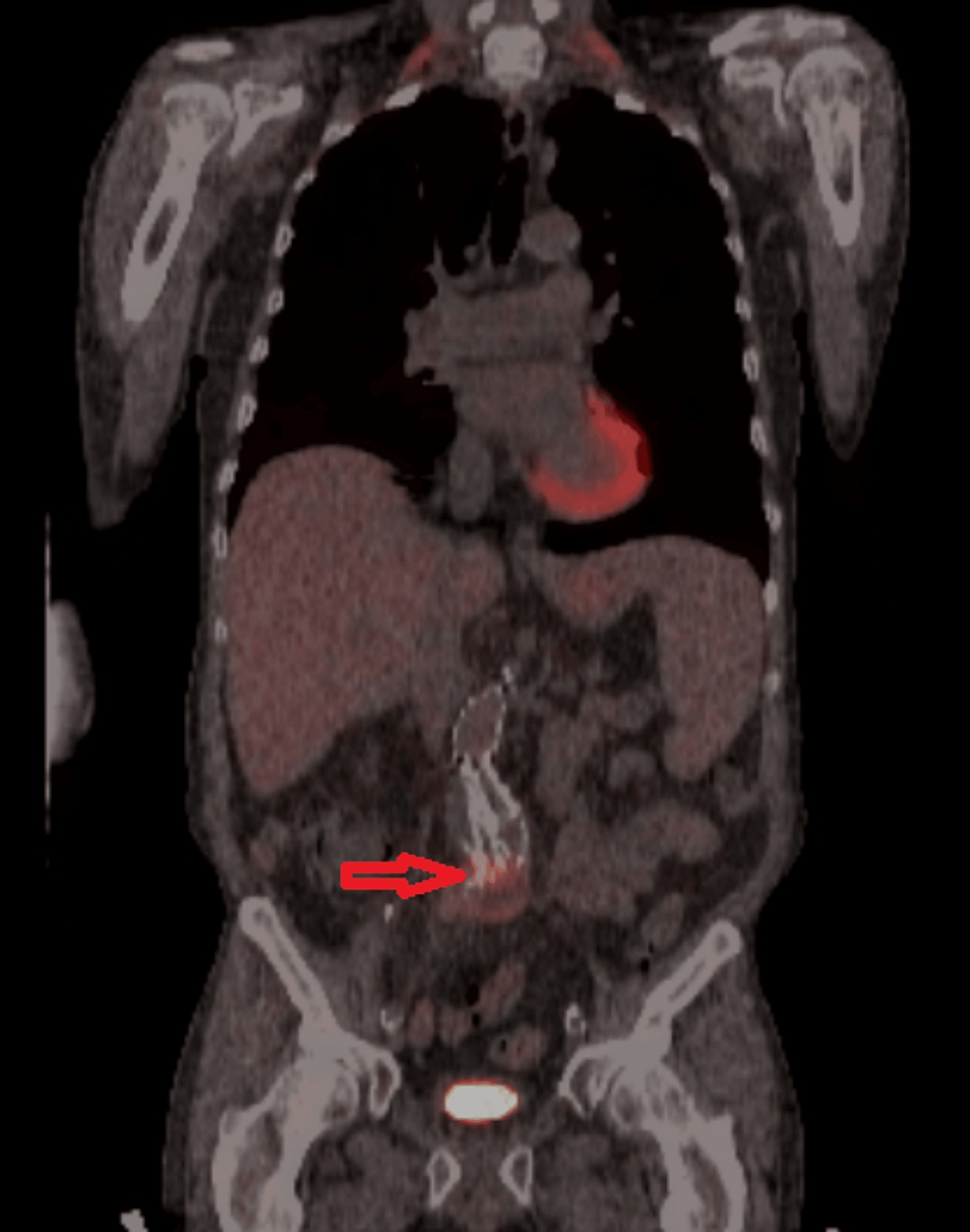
Heterogenous fluorodeoxyglucose (FDG) uptake involving the enlarging abdominal aortic endoleak (red arrow) at the site of the previous stenting
